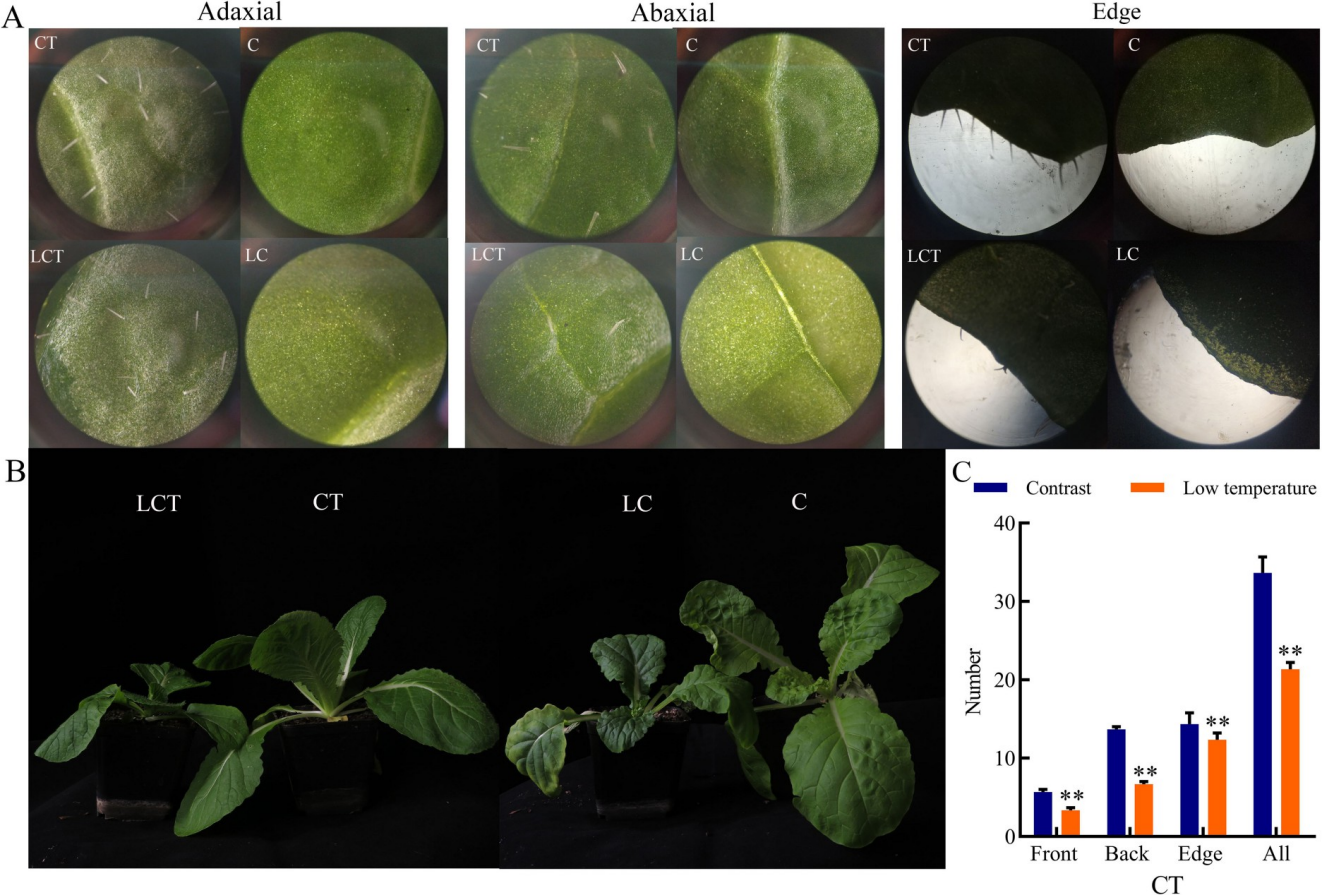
Effect of low temperature treatment on trichomes. A, Three visual fields (adaxial, abaxial, and edge) at room temperature (CT, C) and low temperature (LCT, LC); B, Plants at room temperature (CT, C) and low temperature (LCT, LC); C, Density of trichomes at room temperature (CT) and low temperature (LCT).
